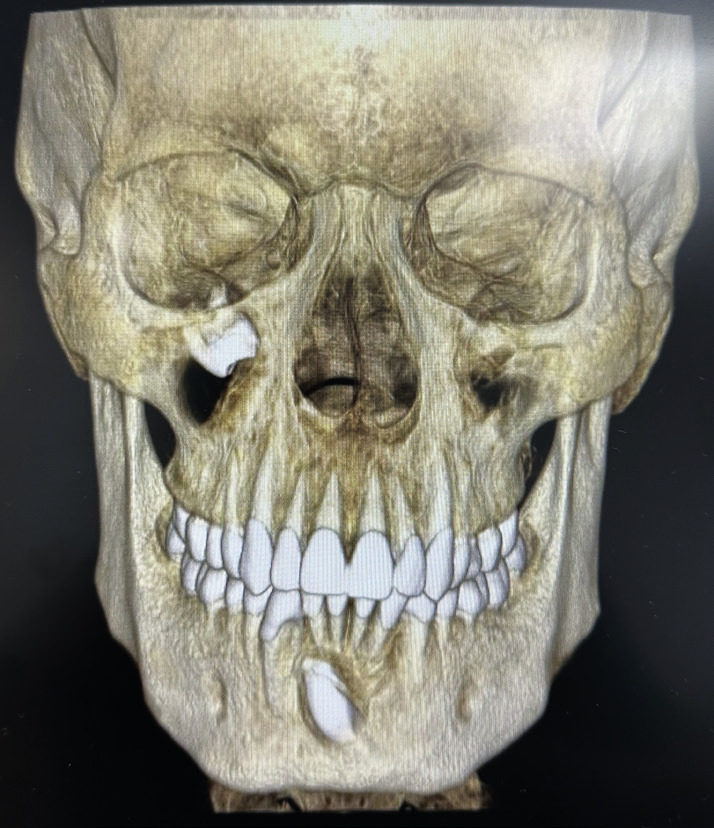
3D image of the CT SCAN showing the ectopic wisdom tooth located in the right orbital floor, within the maxillary sinus.
